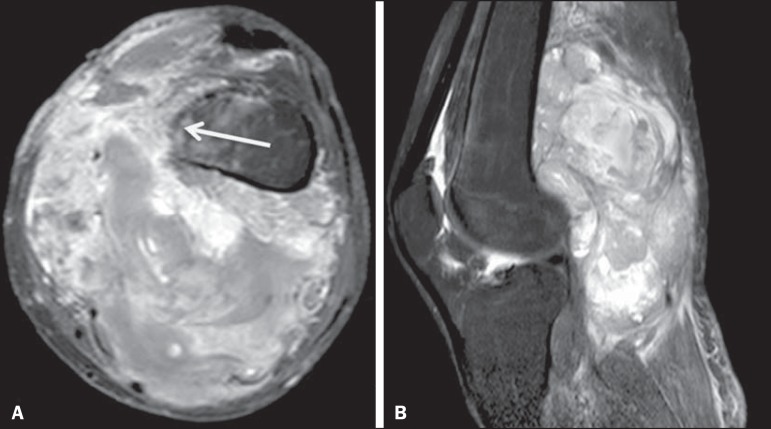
Synovial sarcoma. Fat-suppressed T1-weighted MRI sequences in the axial
